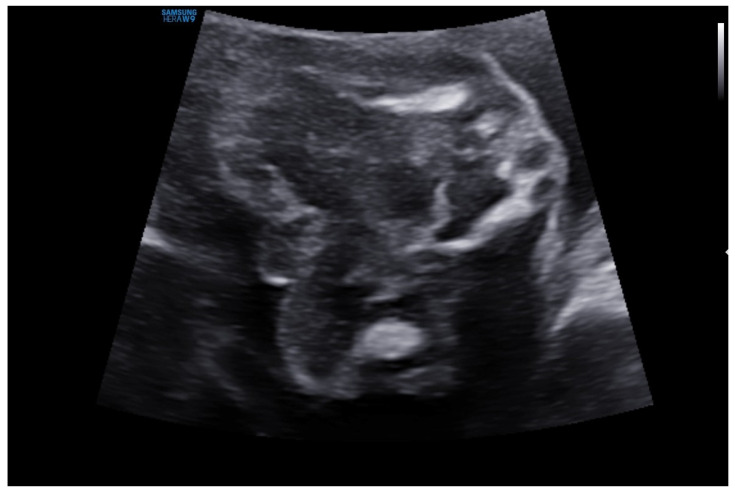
Ambiguous genitalia observed during an ultrasound examination at 20 weeks of pregnancy.
